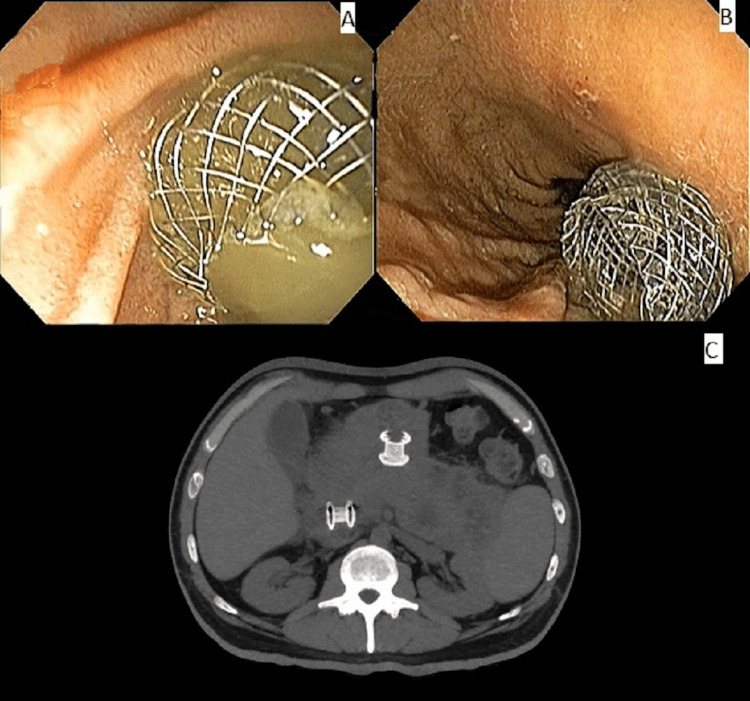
Endoscopic and computed tomography view of the two lumen-apposing metallic stents. (A) Transduodenal stent. (B) Transgastric stent. (C) Control abdominal computed tomography.
